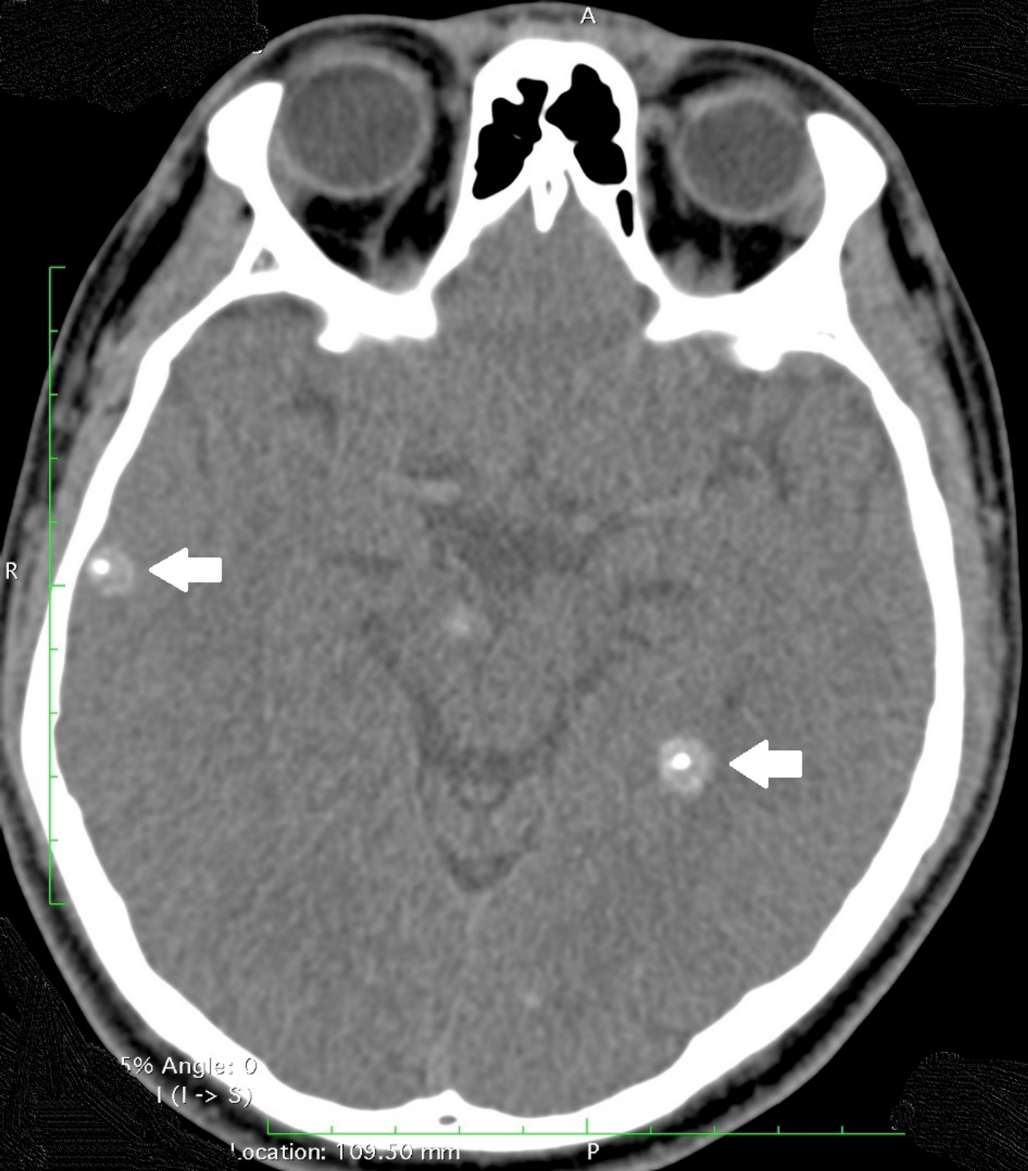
Plain CT of the brain, axial cut, showing calcified scolex within the cyst (thick arrow), giving a “target appearance.”
